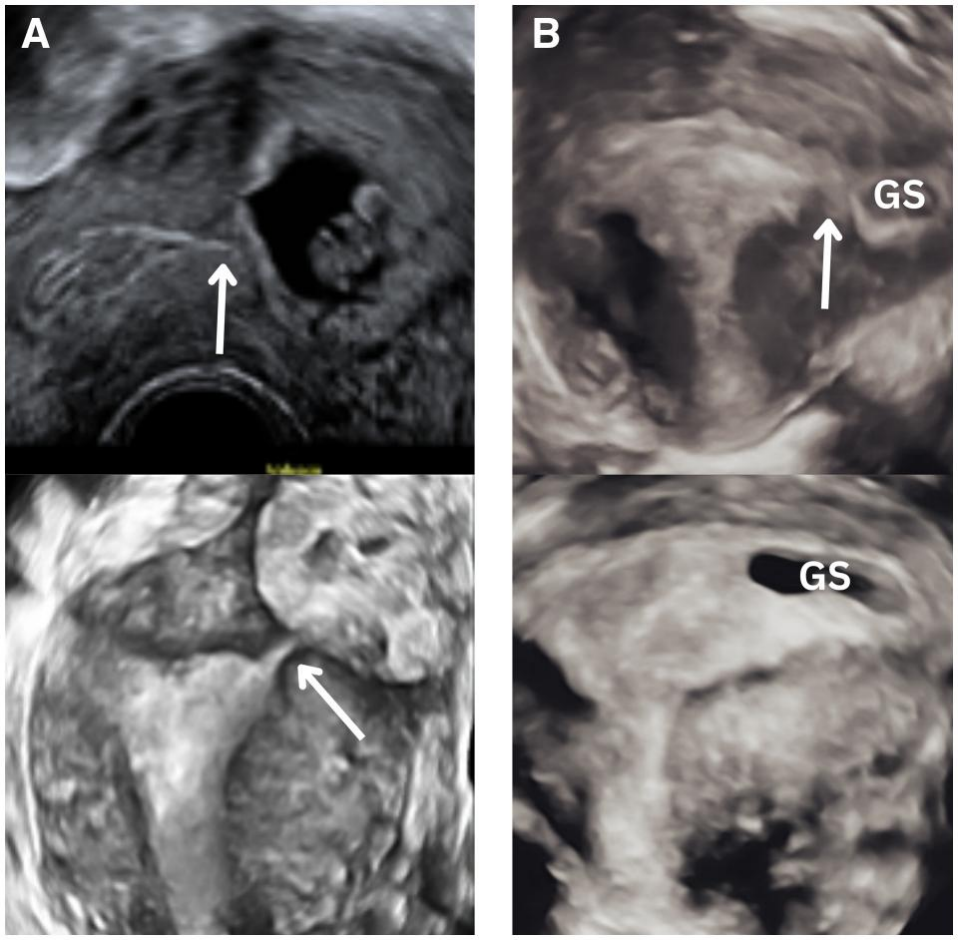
Ultrasound images comparing complete interstitial ectopic pregnancy with a laterally located eutopic pregnancy. (A) The gestational sac (GS) of a complete interstitial pregnancy, with the interstitial line demonstrated on both 2D and 3D ultrasound images by the white arrow. In (B), a laterally located eutopic pregnancy, 3D images demonstrate a laterally located eutopic pregnancy 1 week apart: in the top image the connection to the cavity (demonstrated with a white arrow) is not clear, leading to concern regarding potential interstitial location. However, as the GS expands, it is clear that it is eutopic. In cases of diagnostic uncertainty, repeating the scan at an interval is recommended to minimize the risk of false-positive diagnosis.
